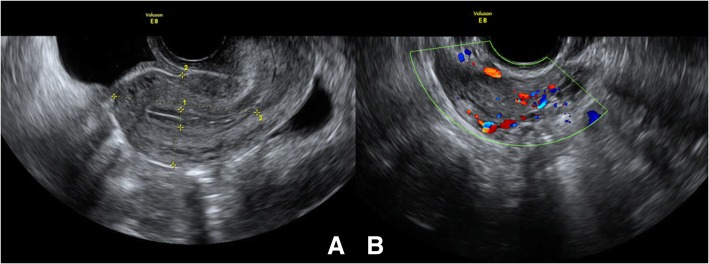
Postoperative images: a and b Transvaginal color Doppler ultrasonography. a uterus, median sagittal section; b The right ovary has complete shape and good blood supply
